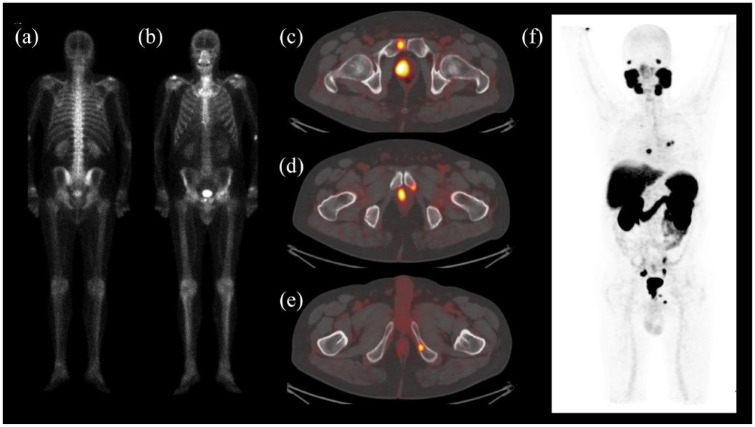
Bone scan (a, b) and [^68^Ga]PSMA PET/CT (c–f) performed for primary staging of a 64 year old patient with prostatic adenocarcinoma, Gleason score = 4 + 5, PSA = 10.1 ng/ml. The bone scan shows a small area of uptake at the right pubic bone (b). Fused transaxial images (c–e) and MIP (f) of the PSMA PET/CT scan confirm the right pubic bone lesion (c, f) and show further PSMA-positive bone lesions with intense PSMA uptake in the left pubic bone (d, f) and left ischio-pubic ramus (e, f). CT, computed tomography; MIP, maximum intensity projection; PET, positron-emission tomography; PSA, prostate-specific antigen; PSMA, prostate-specific membrane antigen.
